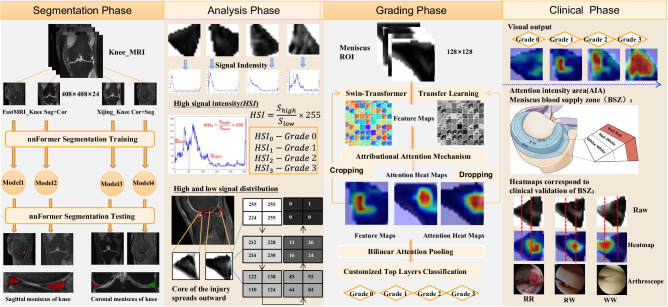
The overall framework of the functional module. The figure summarizes four aspects of this paper, the first part is the segmentation task of meniscus region from the entire knee MRI, the second part is the analysis of meniscus signals, the third part is the fine-grained grading task of meniscus injuries, and the fourth part combines its results with clinical knowledge to propose a new subtype classification and verify its results.
